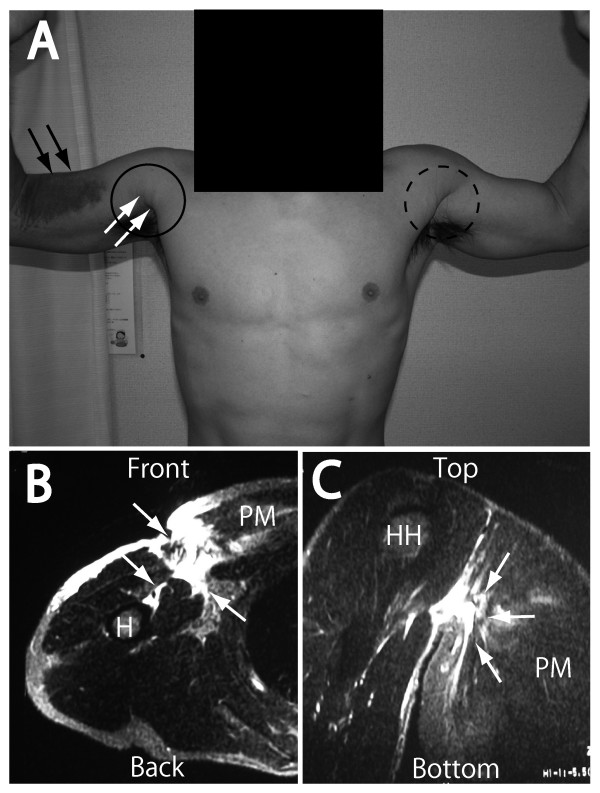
Diagnosis of acute tendon tears of the pectoralis major muscle in patient 2. Clinical photograph of the patient shows ecchymosis over the anterior aspect of the right arm 2 days after injury (A, black arrows) and loss of the axillary fold (A, white arrows inside the black circle; the dotted black circle indicates the normal left side). Magnetic resonance imaging (MRI) with axial (B) and coronal (C) views; spin echo T2-weighted image shows disruption of the tendon of the pectoralis major (PM) muscle. The area of high signal intensity has a fluid-fluid level (B and C, white arrows), which was thought to indicate a loculated hematoma. PM, pectoralis major; H, humerus; HH, humeral head.
